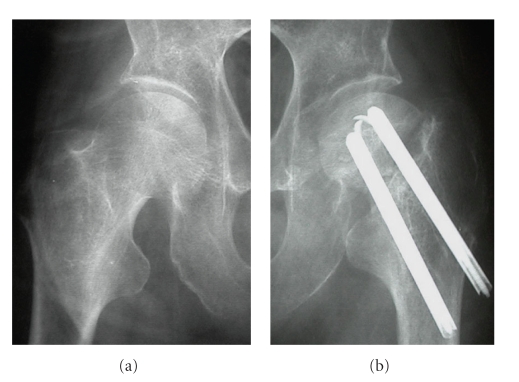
Radiographs show irregularity of the surface of the left acetabulum and osteoporotic changes of the superior part of the left acetabulum in comparison with the right hip. There is an obvious pseudoarthrosis of the femoral neck after internal fixation.
